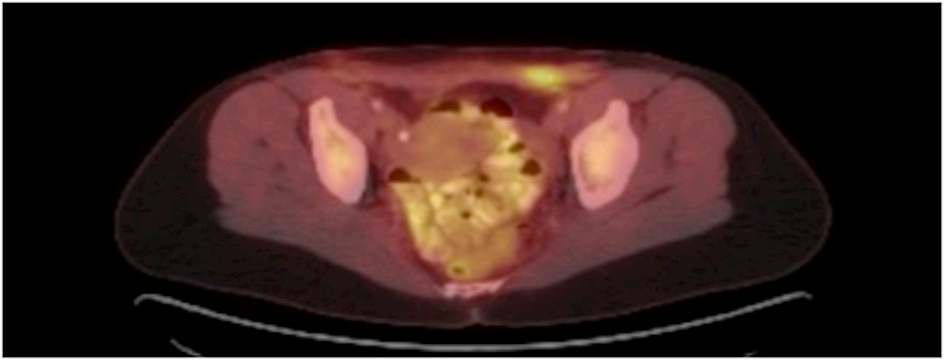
PET CT with hypermetabolic activity with abdominal wall soft tissue stranding and pelvic/inguinal hypermetabolic lymphadenopathy.
